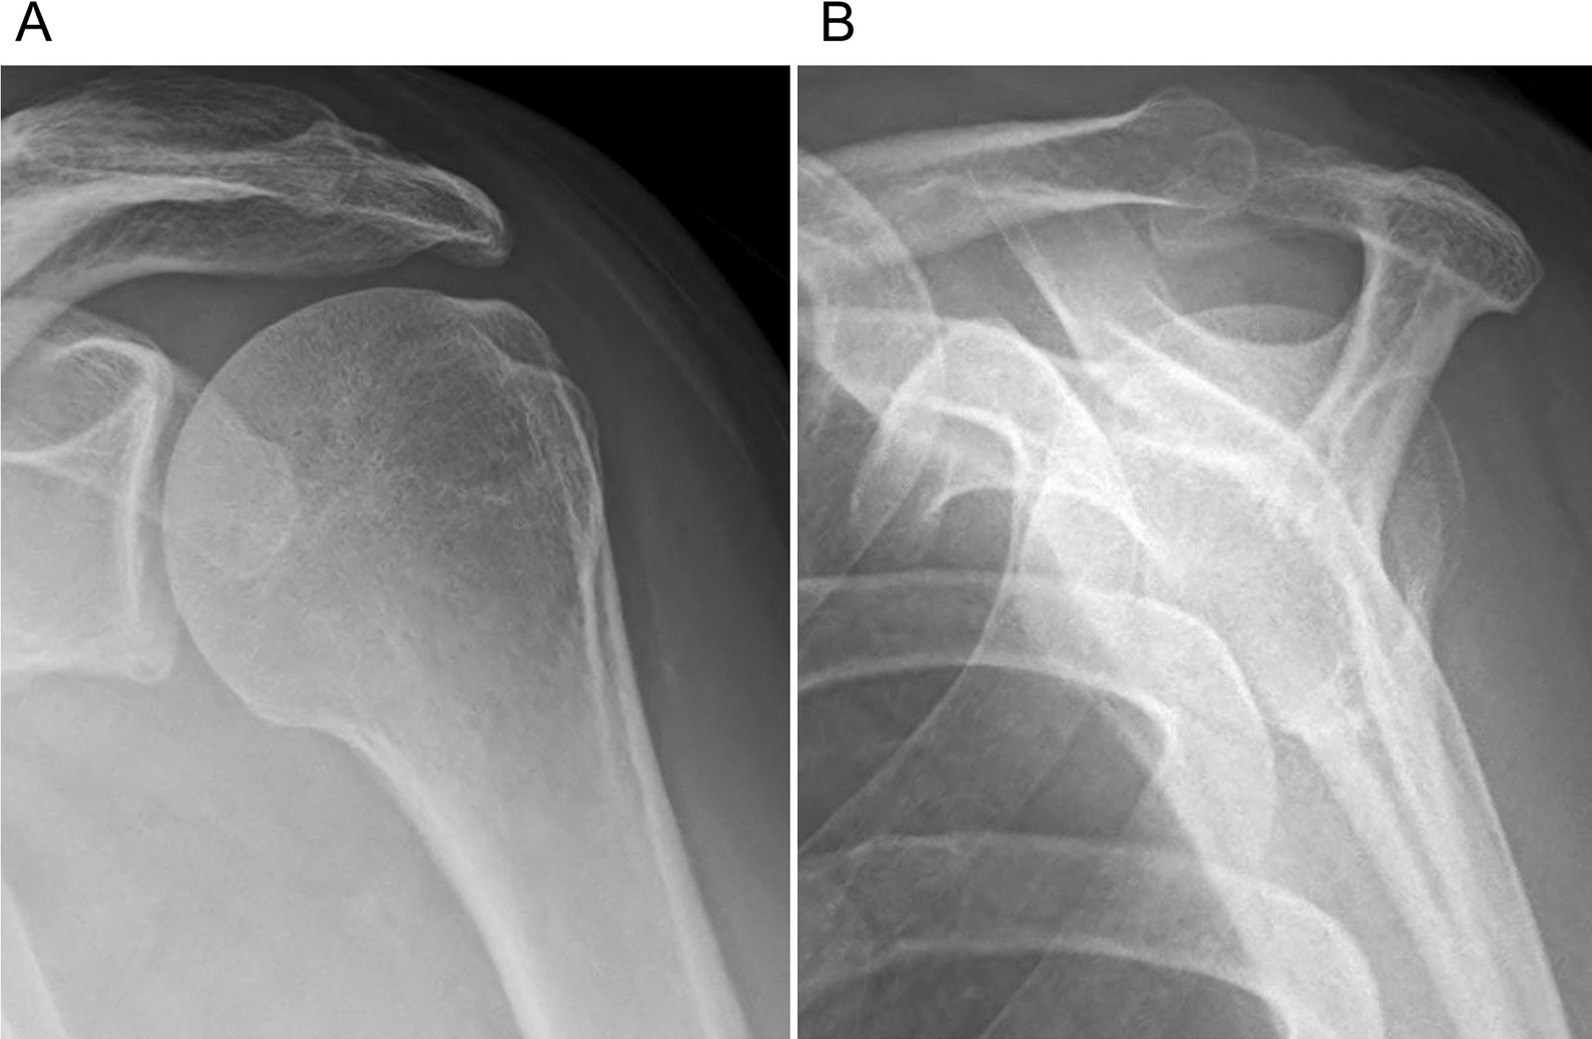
Radiographs of the left shoulder at the initial visit. A Anteroposterior view, B scapular Y view
